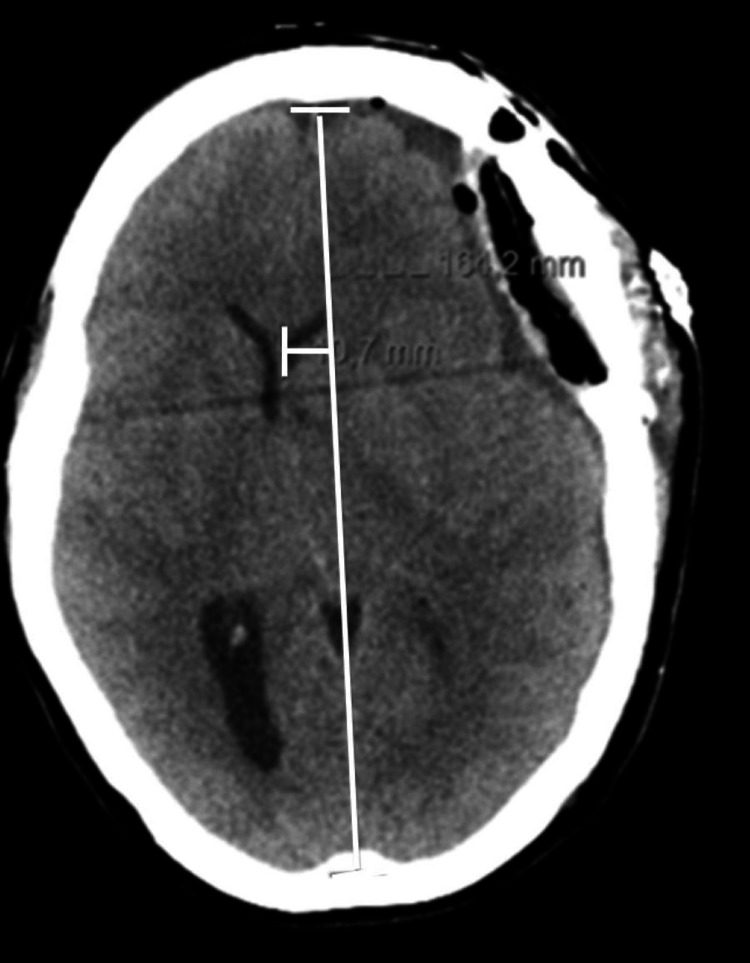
Axial plane of brain computed tomography at the third post-operative day and 24 hours under sedation A pericerebral hypodense collection remains, currently extending to the high fronto-parietal convexity. Consequently, there is a greater deformation of the brain parenchyma, resulting in a deviation of the midline to the right, currently approximately 11 mm, a degree greater than that shown in the previous examination (Figure 2).
